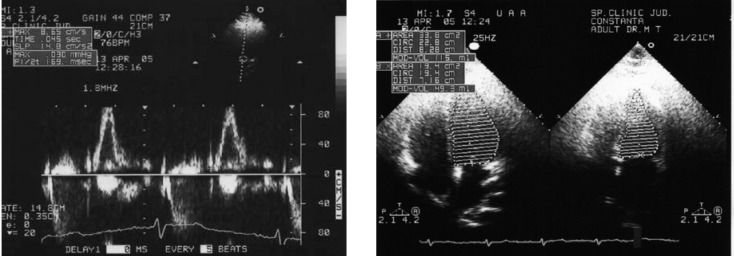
Echocardiography: left ventricular mass index, fractional shortening and fiber shortening velocity improved after 12 month of low-dose therapy with re-hGH.
